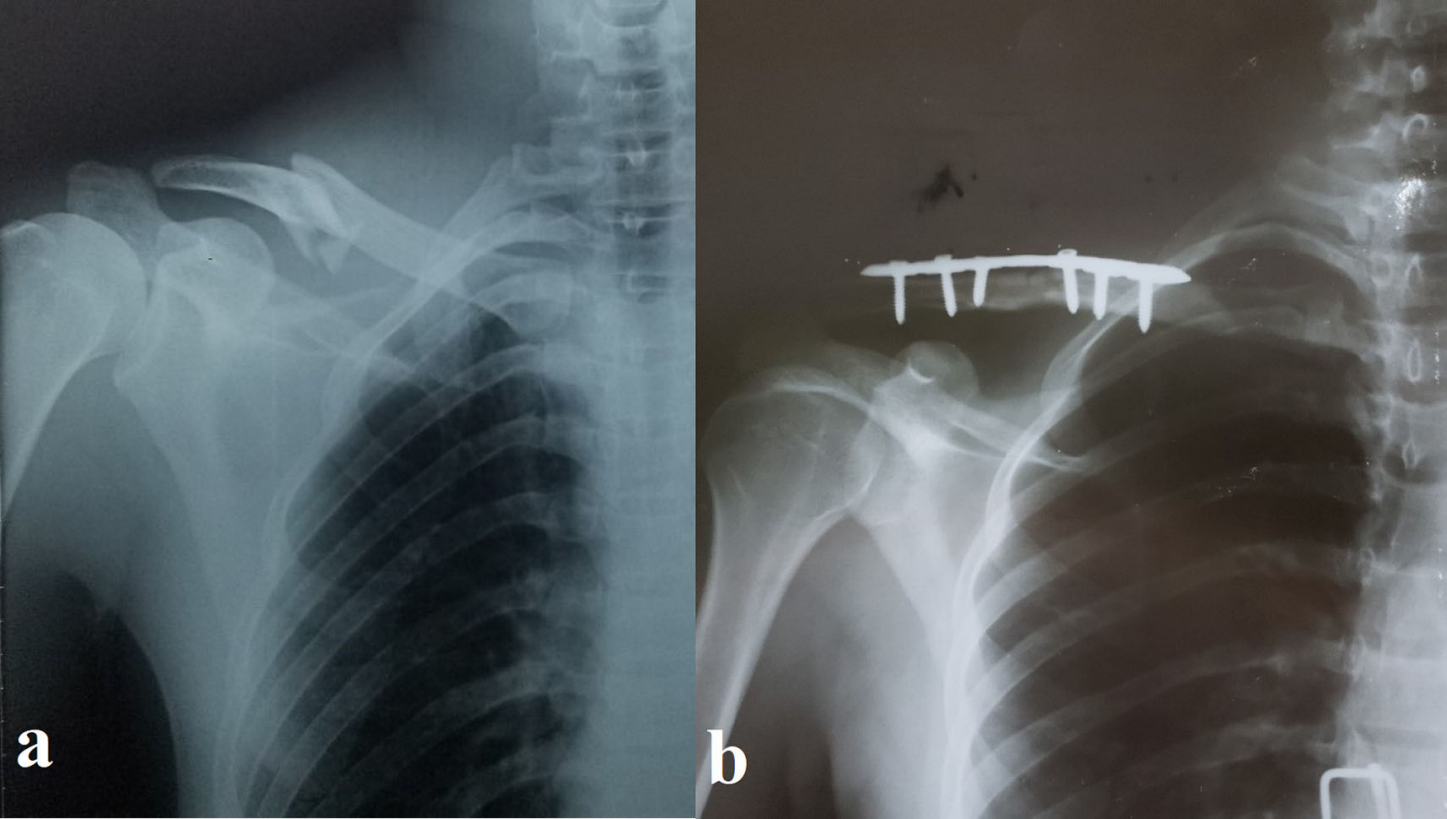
Pre- and postoperative radiographs of a 30-year-old male with displaced midshaft clavicle fracture. (a) Preoperative anteroposterior radiograph showing a displaced, slightly comminuted midshaft fracture of the right clavicle in a 30-year-old male patient. (b) Postoperative anteroposterior radiograph of the same patient after open reduction and internal fixation (ORIF) with a precontoured superior locking plate (Orthomed E, 7-hole, titanium). Note the anatomical reduction of the fracture and the use of locking screws that engage at least four cortices on either side of the fracture.
